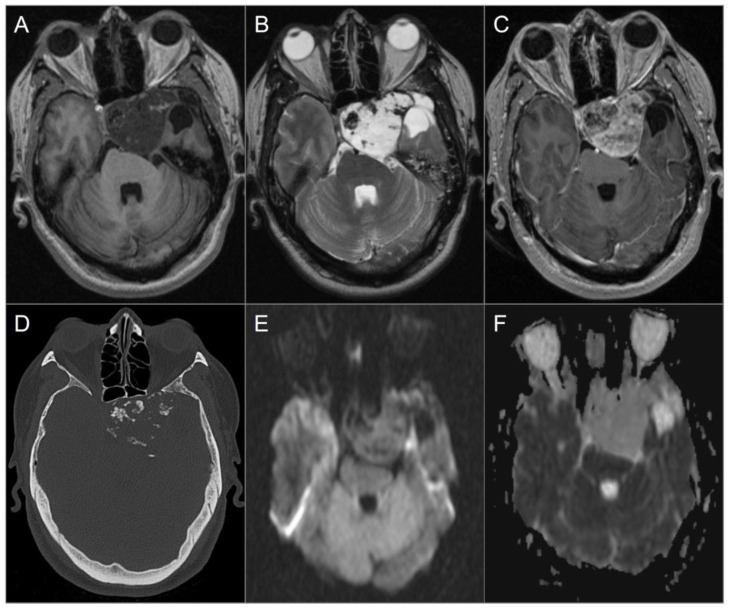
Chondrosarcoma. (A) Axial noncontrast T1W and (B) T2W images show a well-circumscribed T1 hypointense, markedly T2 hyperintense lesion centered in the left petroclival fissure involving the clivus, cavernous sinus, posterior aspect of the sphenoid sinus and left middle cranial fossa. The lesion extends into the prepontine cistern and compresses the brain stem, left temporal lobe, and basilar artery. (C) Axial postcontrast T1W image shows avid heterogeneous enhancement of the mass. (D) Axial CT image shows permeative destructive margins and chondroid calcifications in the lesion. (E) DWI and (F) ADC map images reveal facilitated diffusion in the lesion.
